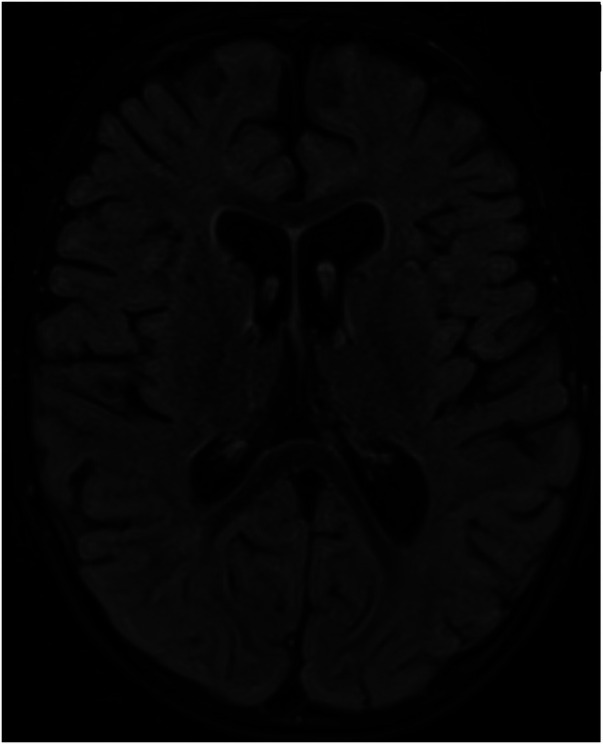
Brain MRI acquired at 19- months of age after an episode of cardiac arrest showed no evidence of hypoxic ischemic insult on the axial FLAIR image; however, there is evidence of mild brain volume loss with enlarged ventricles and prominent subarachnoid spaces.
